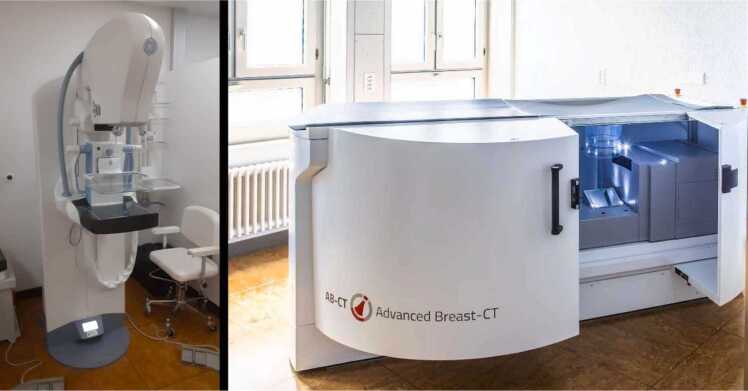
Devices used in this study. Left: conventional mammography (Senographe Essential GE Healthcare). Right: photon-counting spiral BCT (nu:view, Advanced Breast-CT GmbH).
